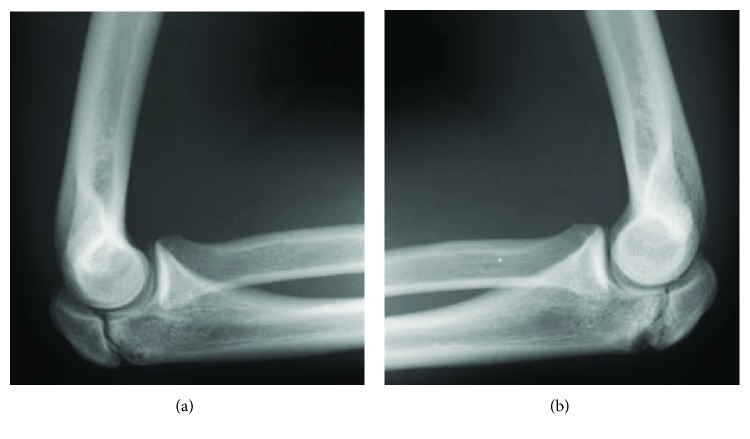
Radiographs of the elbows of a 15-year-old male patient (Patient 1). Lateral radiograph of both elbows showing delayed union of the olecranon apophysis. (a) The right elbow. (b) The left elbow.
